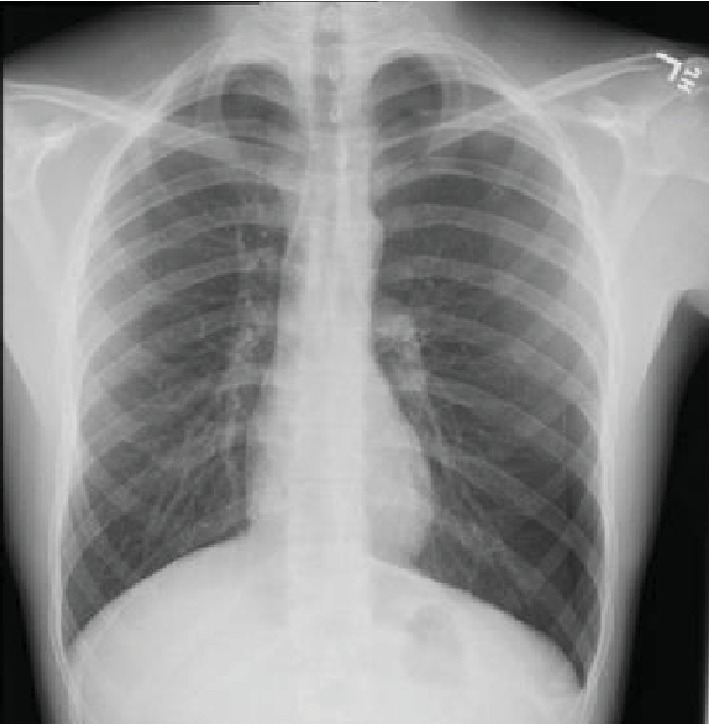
Chest X-ray showing moderate sized left-sided pneumothorax.
